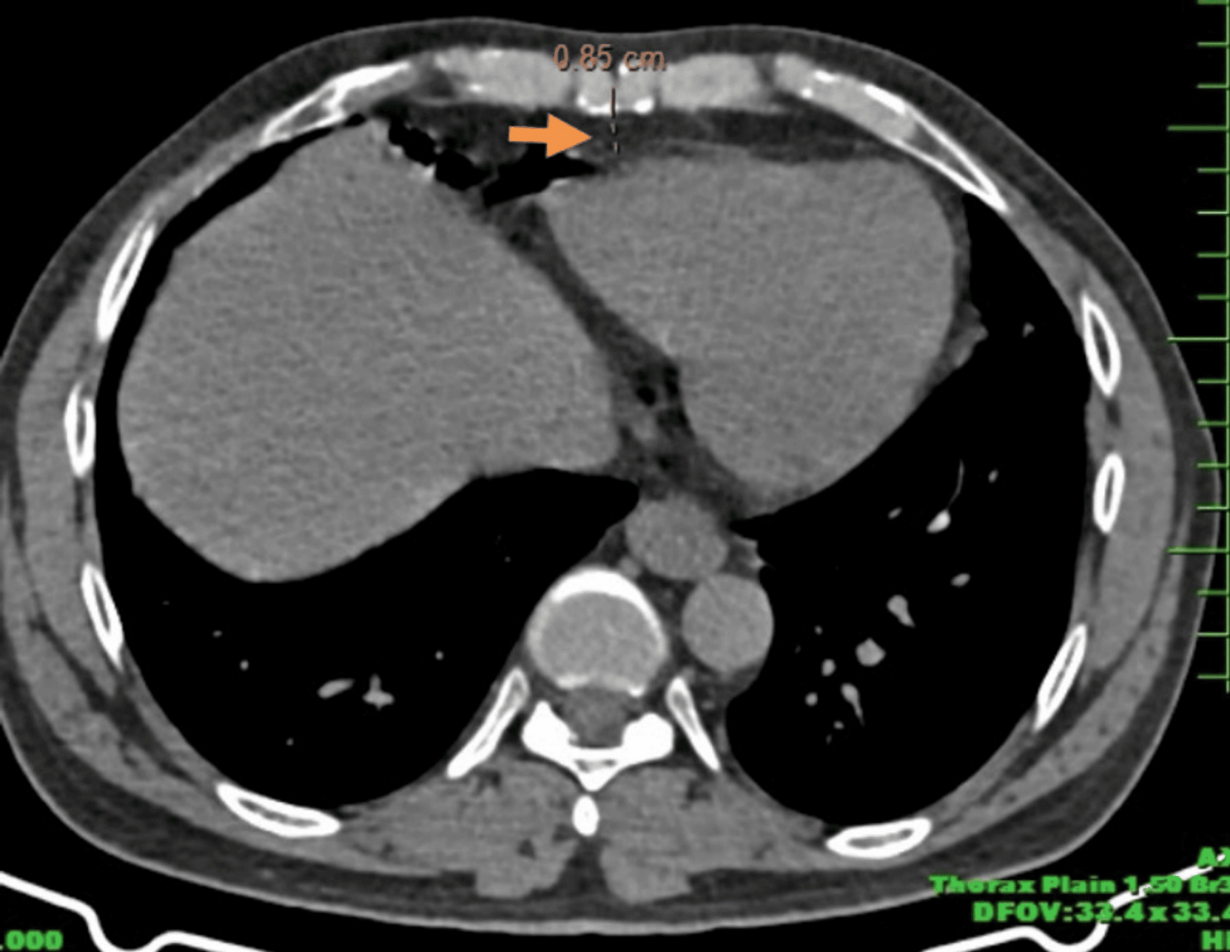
Axial section of CT thorax image showing the measurement of pericardial fat pad thickness (orange solid arrow)
